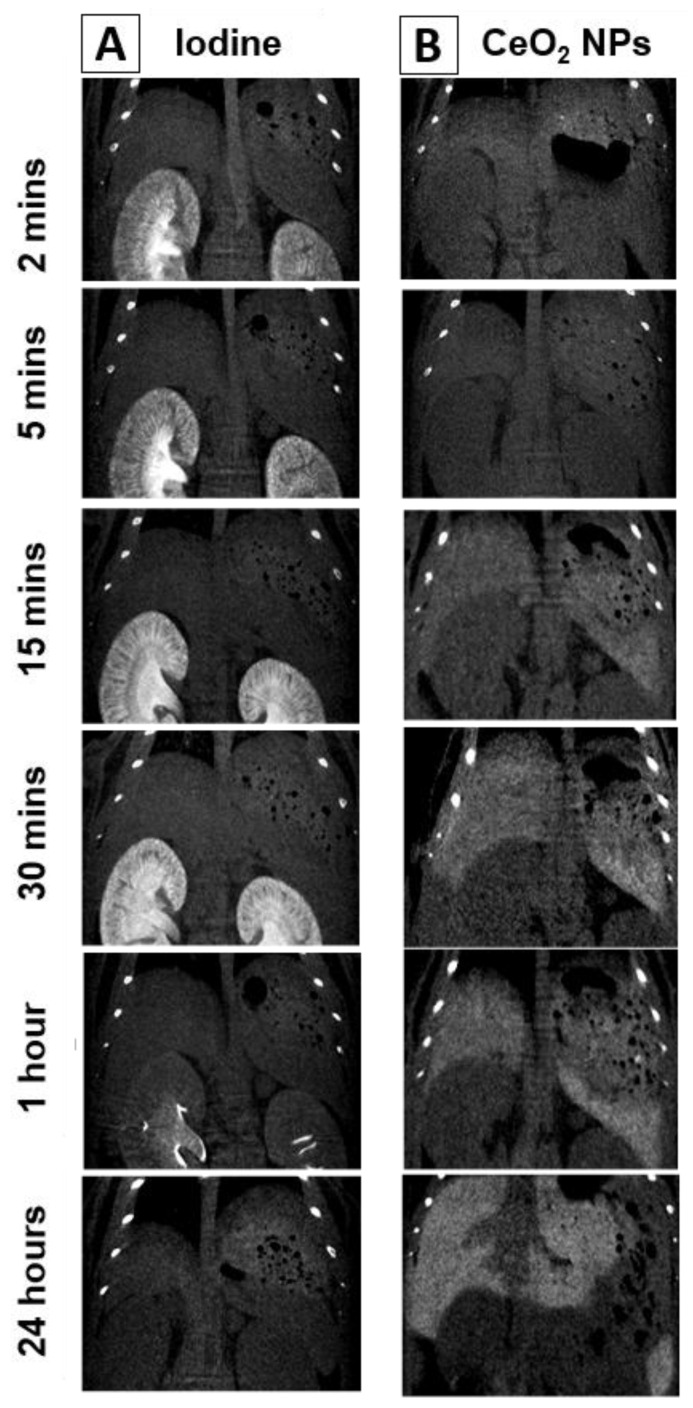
In vivo coronal CT images after intravenous injection of (A) the commercial iodine contrast agent Iopamidol^®^-370 (150 µL; 175 mg I/mL) and (B) CeO_2_NPs-MSA solution (200 μL, 10 mg Ce/mL) at various representative times, from 2 min post-injection up to 24 h.
